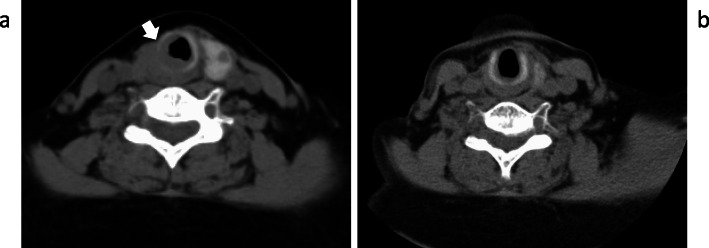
CT findings before and after radiation. a Stenosis of the trachea occurred due to recurrent tumor invasion (arrow). b The recurrent tumor could not be recognized 1 year after radiation therapy.
